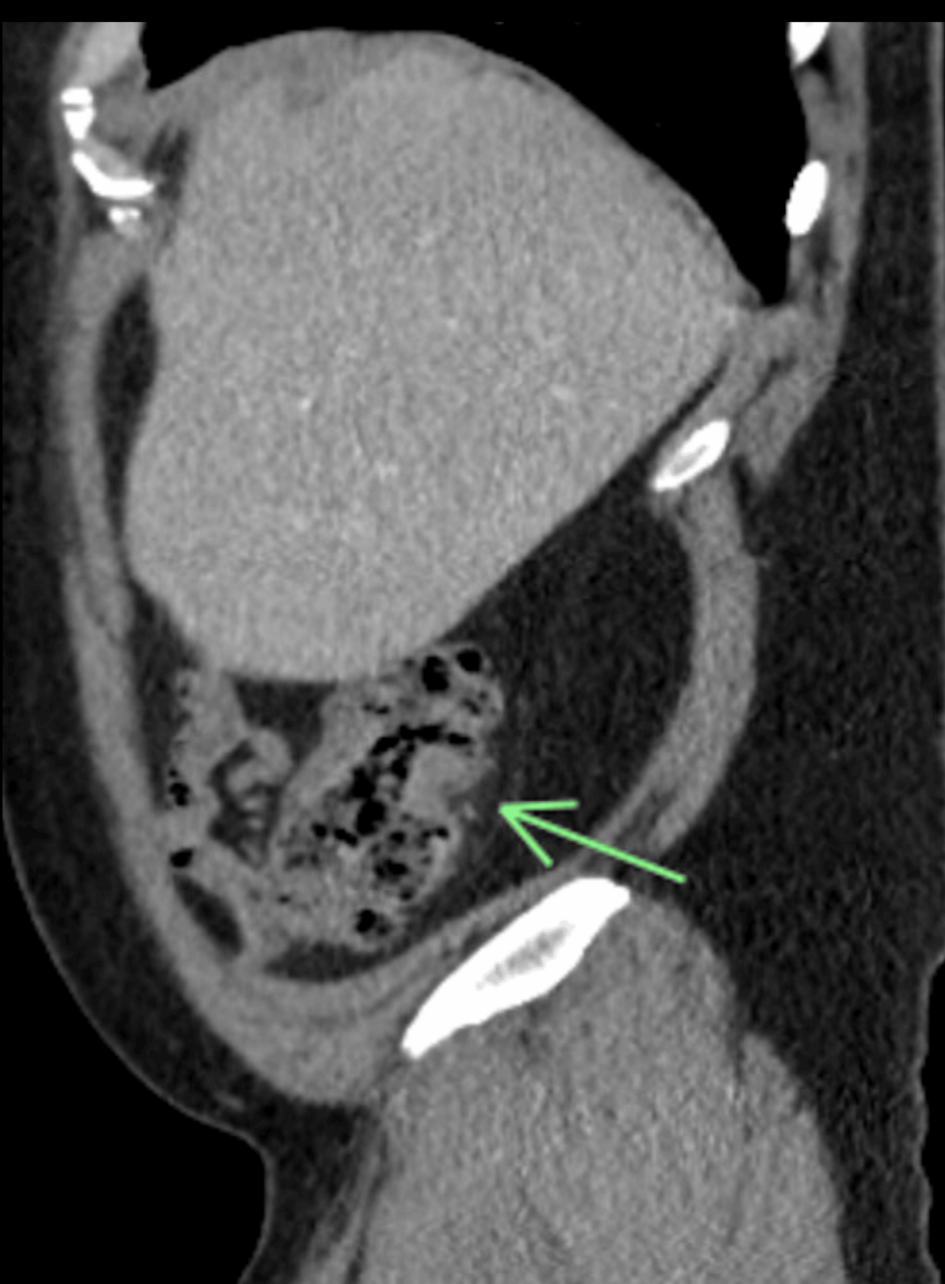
CT scan of the abdomen showing ascending colon stricture Arrow showing localized wall thickening and luminal narrowing of the ascending colon with surrounding inflammation
